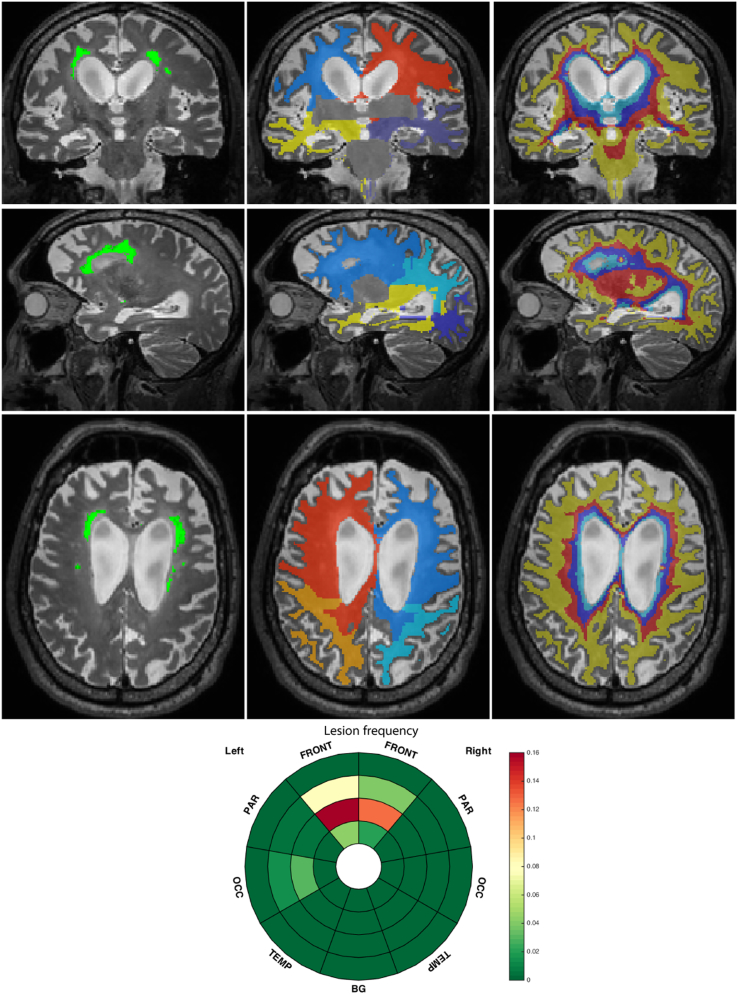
Example of the location patient-specific scheme for the three orientations. From left to right: 1st column: T2-weighted image with overlayed lesion segmentation; 2nd column: lobar separation; 3rd column: layer discretisation. The corresponding lesion frequency per zone is given in the inset image at the bottom.
